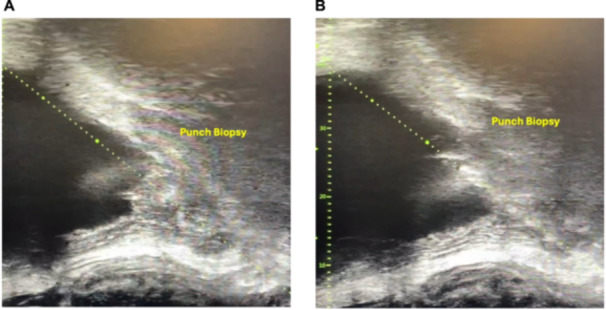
Micro‐ultrasound guided prostatic fossa biopsy targeting a recurrent lesion in the prostatic fossa before (A) and after (B) needle placement. [Color figure can be viewed at wileyonlinelibrary.com]
